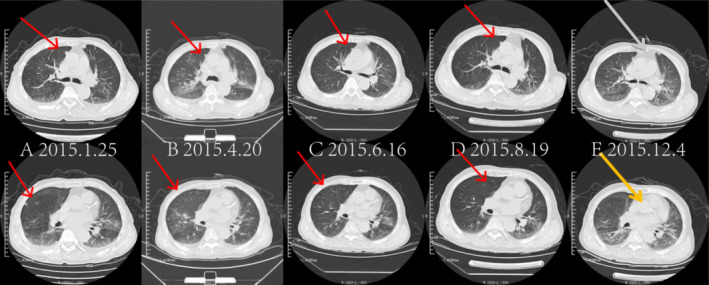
Changes in lung lesions from January 2015 to December 2015: The number of lung metastases significantly decreased, with a substantial reduction in volume and continuous improvement in lesions. Panels A–E show plain chest CT scans of lung tumor metastases, which were nearly in the same plane, gradually decreasing and eventually disappearing between January 25, 2015, and December 4, 2015 (arrows).
